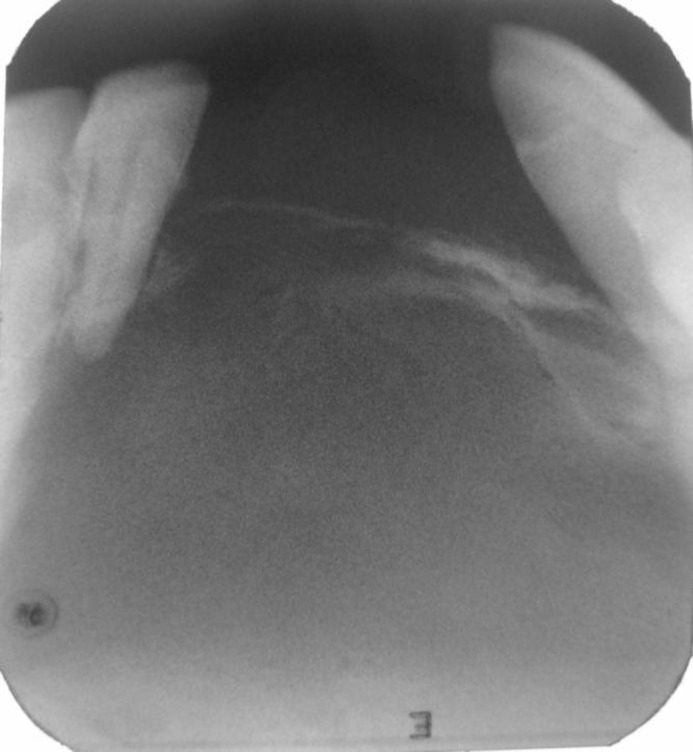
Periapical radiography: border is not intact in some areas.
